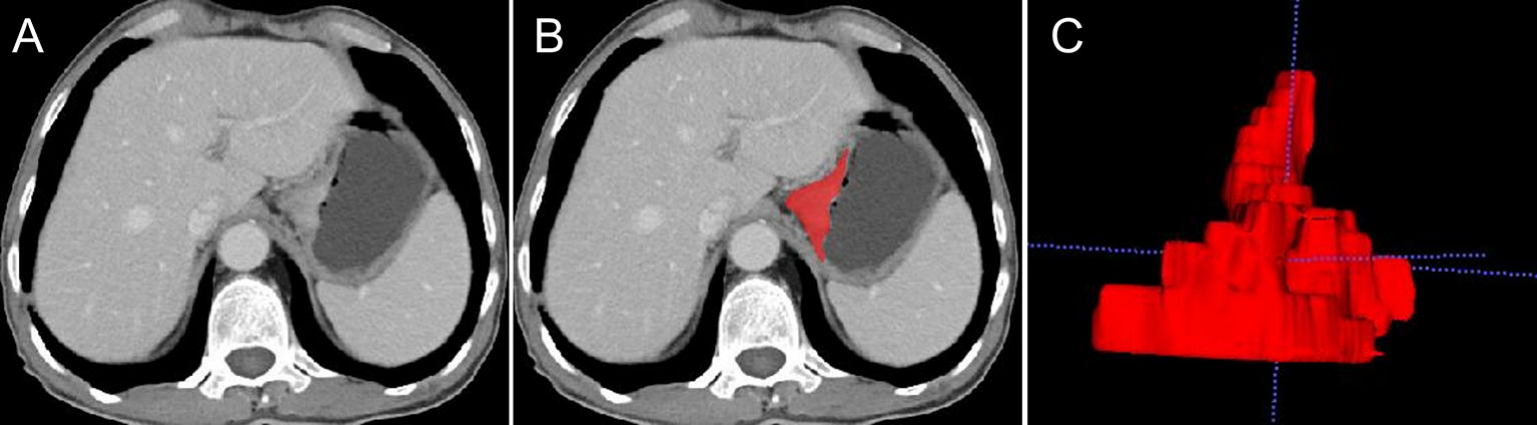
An example of manual segmentation of EGJ adenocarcinoma. (A) A localized mass with heterogeneous enhancement is observed at the EGJ on the venous-phase CT image. (B) Segmentation of the same axial slice. (C) 3D volumetric reconstructions of the tumor generated from ITK-SNAP.
